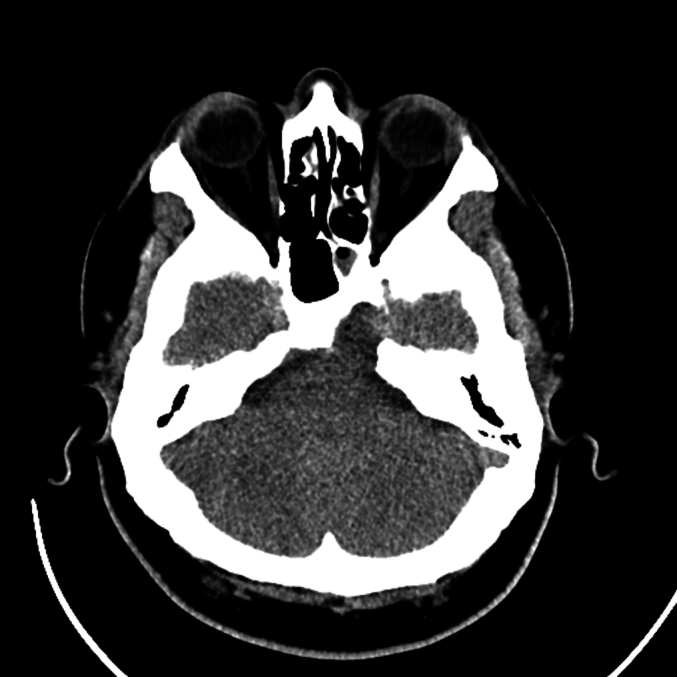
Pre-operative axial CT scan revealing a hypodense lesion in the hypothalamic region and proximal pituitary stalk, with no significant involvement of surrounding bone structures.
